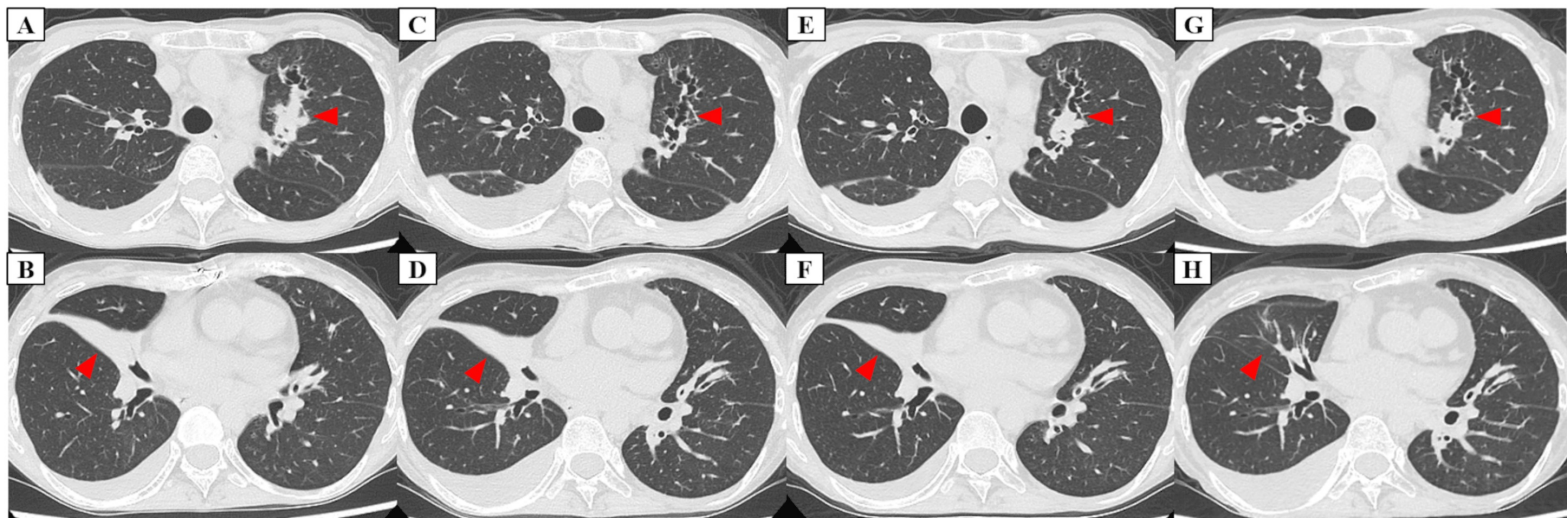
Serial chest CT changes during mepolizumab and after switch to tezepelumab. At the start of mepolizumab therapy (A, B), chest CT shows central bronchiectasis with segmental mucus plugs in dilated bronchi of the left upper lobe (LUL) and consolidation with atelectasis in the right middle lobe (RML). Twelve months after starting mepolizumab (C, D), the LUL mucus plug burden had decreased. Fifteen months after starting mepolizumab (E, F), LUL mucus plugs recurred. Six months after starting tezepelumab (G, H), the LUL mucus plug burden had further decreased, and the prior RML atelectasis/consolidation had resolved.
